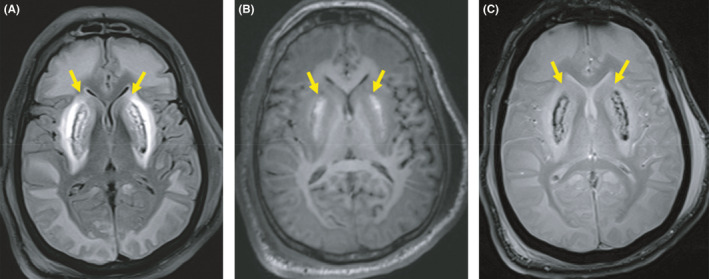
Axial brain magnetic resonance images showing putaminal hemorrhagic necrosis (arrows). (A) FLAIR sequence showing bilateral heterogeneous putaminal hyperintensities surrounded by hypointense borders. (B) T1‐weighted image: high signal on putaminal affected area representing the hemorrhagic nature. (C) Putaminal low signal regions on the T2* sequence related to a hemosiderin component. We note also the diffuse subcortical white matter demyelination (A–C)
